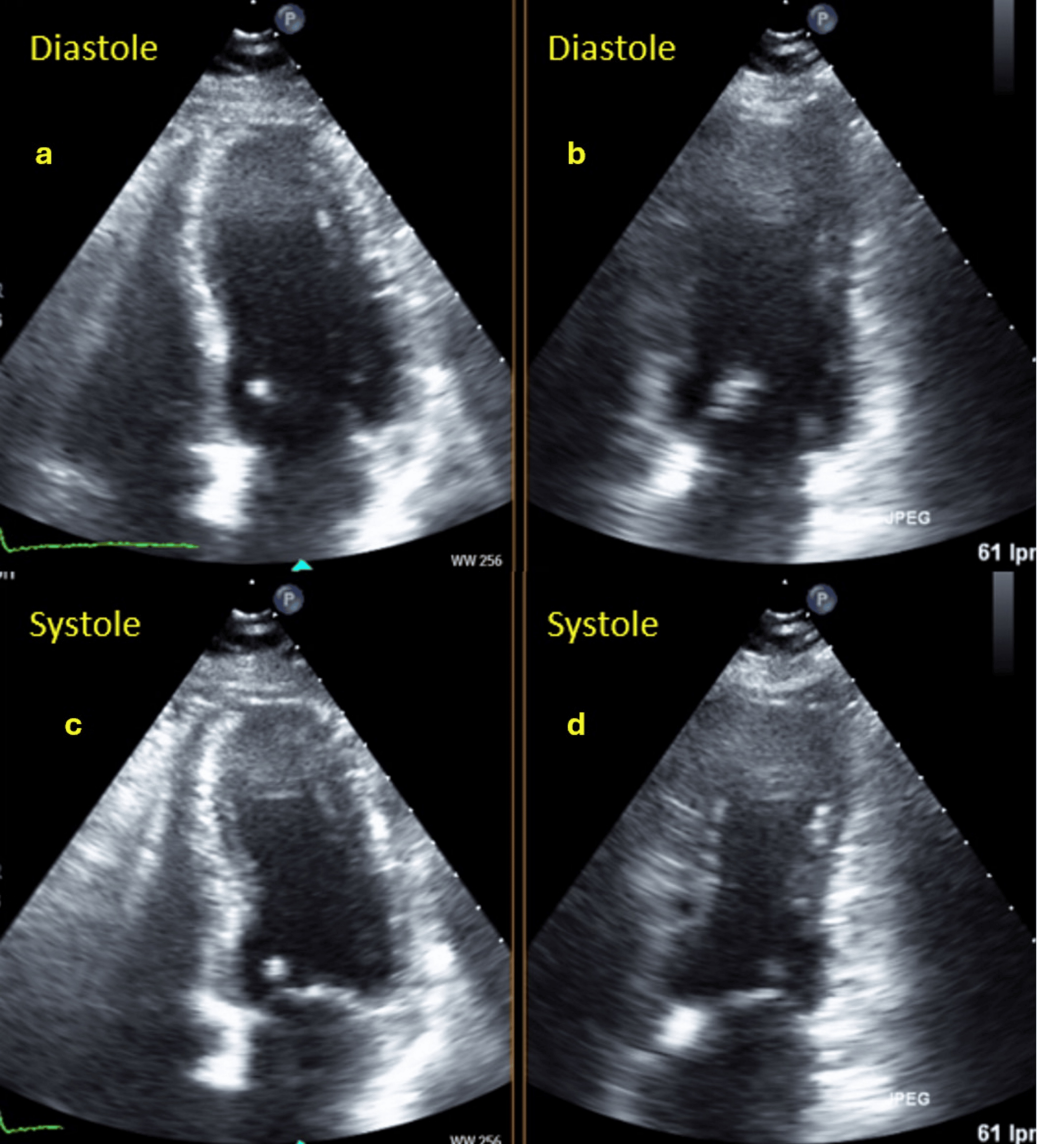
Echocardiogram at discharge shows correction of the alterations in the contractility of the middle segments while maintaining apical akinesia a: LV diastole apical four-chamber view; b: LV diastole apical two-chamber view; c: LV systole apical four-chamber view; d: LV systole apical two-chamber view LV: Left ventricle
